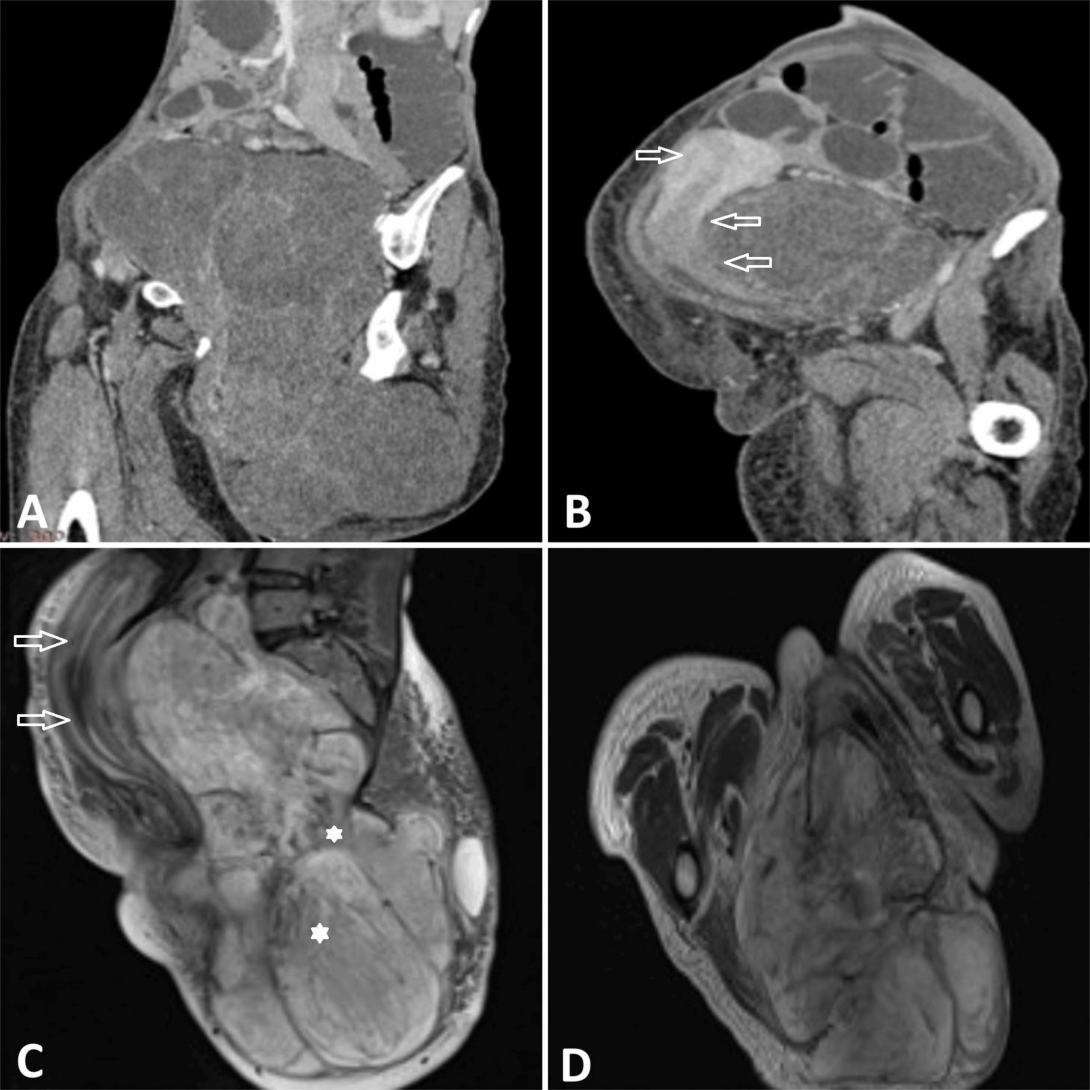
Imaging findings (A & B) CT images show contrast-enhanced sagittal reformated images showing a hypodense mass lesion in the pelvic cavity, perineum, and gluteal region with few foci of septal enhancement within the tumor. The mass lesion is causing anterior displacement of the uterus, cervix, upper vagina, and urinary bladder without any infiltration. (Uterus, cervix and upper vagina marked with arrows from upper to lower). (C & D) MRI images: Sagittal and axial T2 weighted spin echo images show a large high-signal-intensity mass lesion with lower-signal-intensity swirled strands (Indicated by asterisk) within the tumor. The uterus, cervix, upper vagina, and urinary bladder are displaced anteriorly (indicated by arrows).
